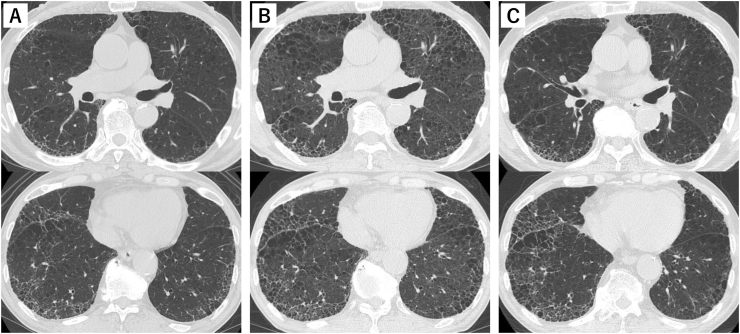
High resolution computed tomography demonstrated emphysema, reticulation, and honeycombing without nodular shadows and mosaic pattern suggesting fibrotic hypersensitivity pneumonitis. (A) Before admission. (B) At admission, the density of lung field increased slightly and diffusely. (C) Two years after addition of oral glucocorticoid, the density of lung field improved.
